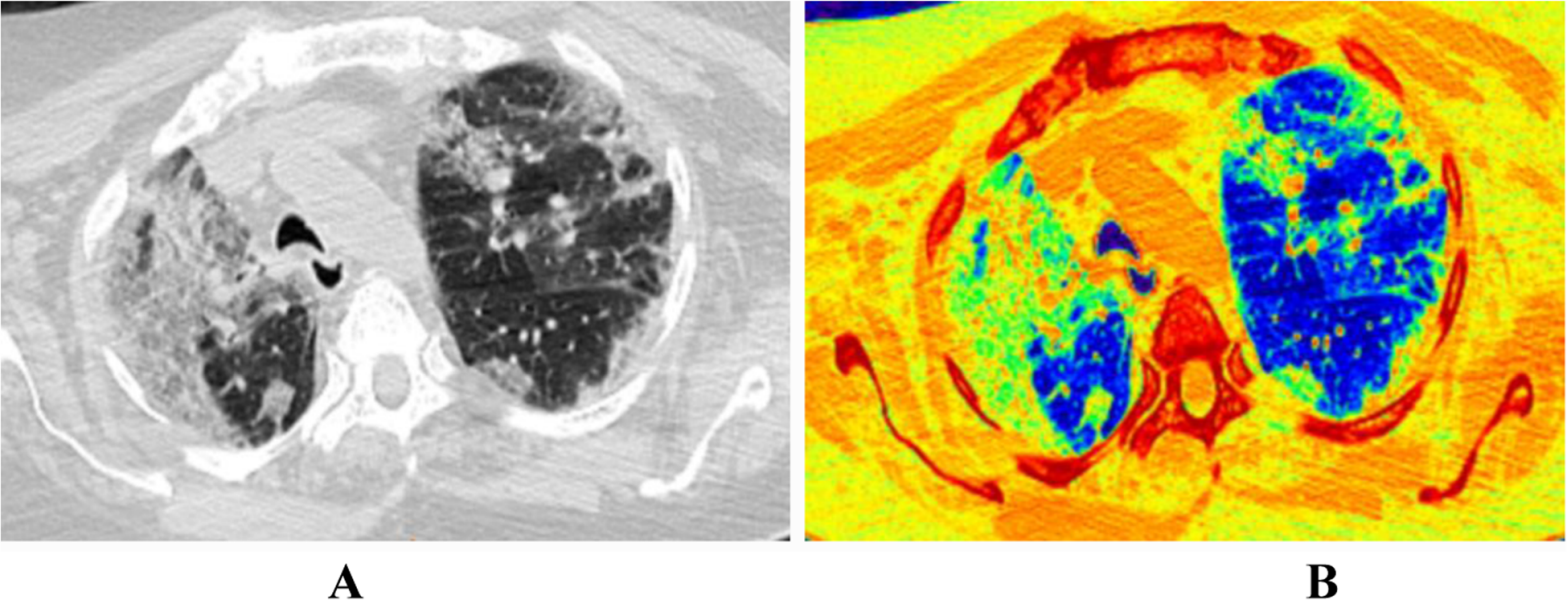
A 55-year-old female patient with first presentation with dyspnea and cough; CT-SSS was 14. Follow-up revealed development of post-COVID syndrome in the form of persistent cough after 60 days from the onset. a MDCT chest, axial cuts show widespread bilateral predominantly peripheral patchy and confluent ground glass opacities with septal and vascular thickening. b Corresponding color coded images
